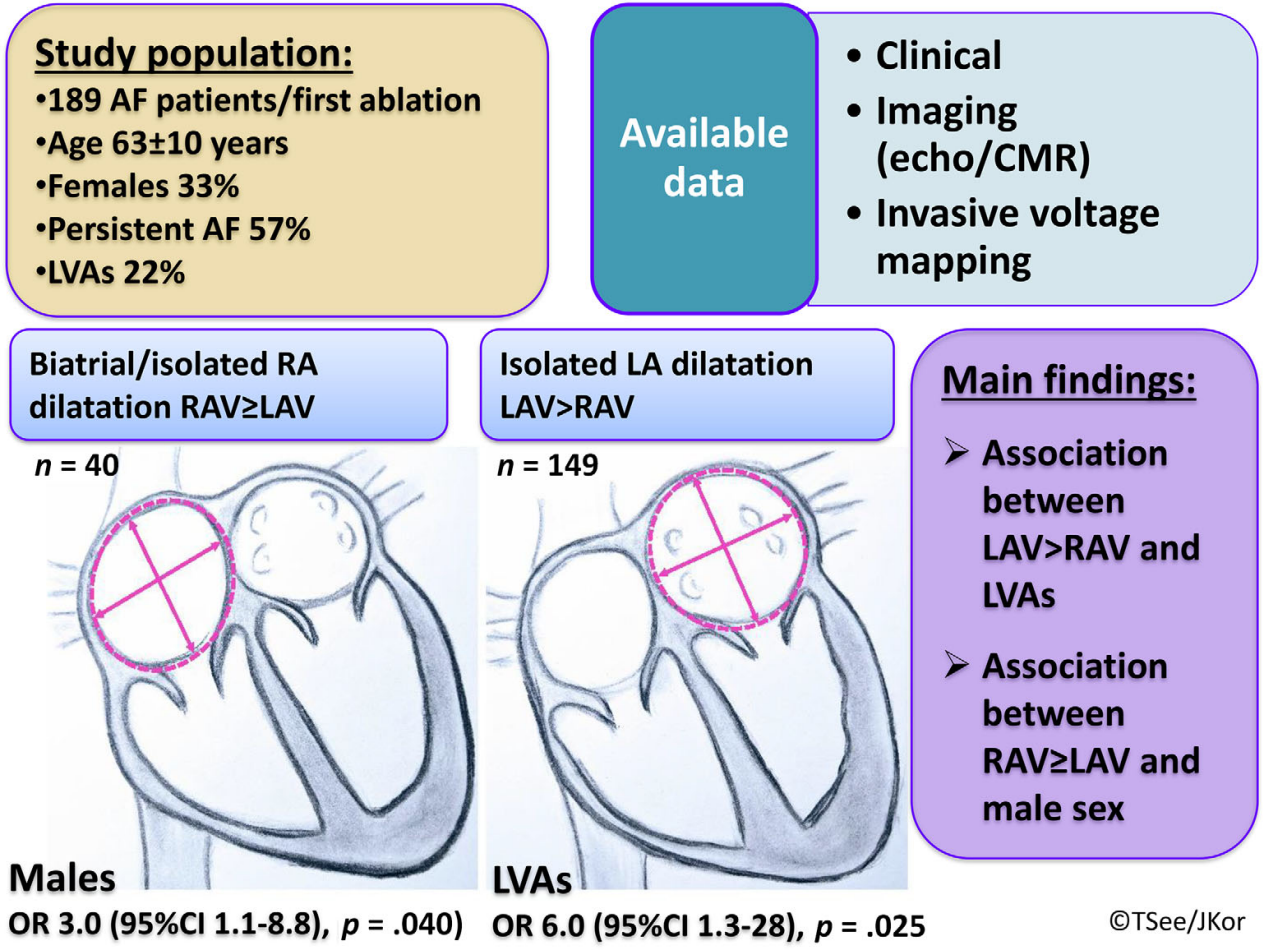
Study overview. Central figure describes the study population and main results. Isolated LA dilatation (LAV > RAV) was significantly associated with LVAs, while biatrial/isolated RA dilatation (RAV≥LAV) was associated with male sex. AF, atrial fibrillation; CI, confidence interval; CMR, cardiovascular magnetic resonance imaging; HR, heart rate; LA, left atrial; LAV, left atrial volume; LVAs, low voltage areas; RA, right atrial; RAV, right atrial volume; OR, odds ratio
